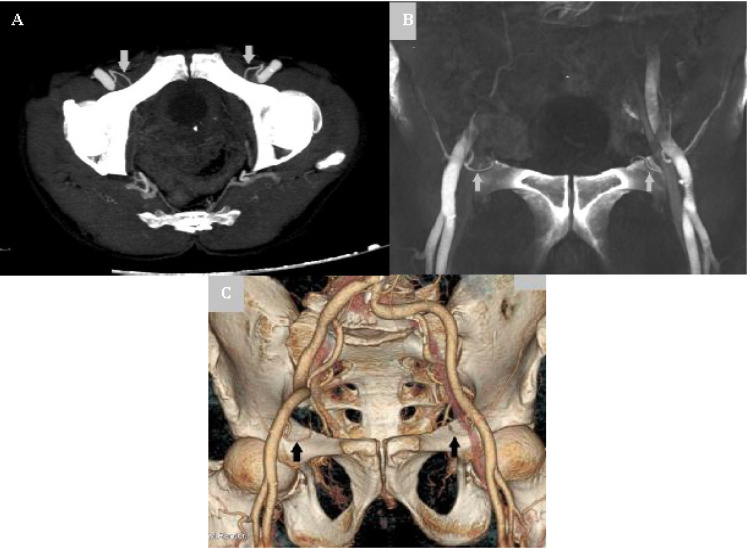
CT angiographic images in a 64-year-old woman showing the bilateral Corona Mortis arteries (arrow). (A) Axial thick MIP (Maximum intensity projection) reconstructed image; (B) coronal shaded surface rendering of the vascular CT study and (C) 3D volume rendered image
